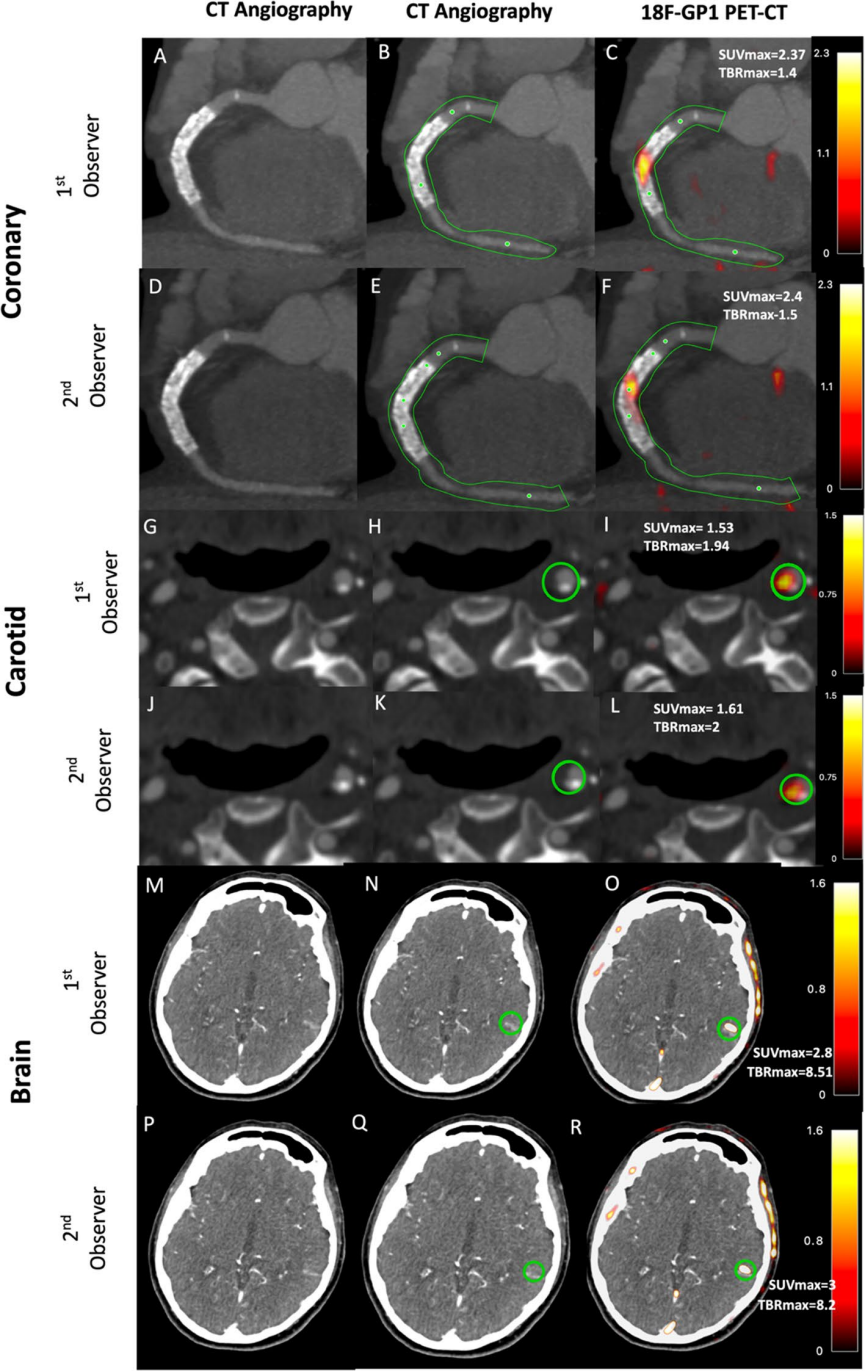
Quantitative ^18^F-GP1 positron emission tomography and computed tomography image analysis. Panels (A)–(F) show a patient presenting with inferior ST elevation myocardial infarct and receiving primary cutaneous intervention to the right coronary artery. CT angiography (A and D) with semiautomated centrelines placed to generate volumes of interest (B and E; green lines representing tubular region of interest). ^18^F-GP1 is seen within culprit right coronary artery and stented segment (C and F). Panels (G)–(L) show a patient presenting with left partial anterior circulatory stroke secondary to left carotid artery atherosclerotic disease. Carotid artery CT angiography (G and J) shows a mixed plaque disease in the internal carotid artery within the region of interest (H and K; green circle representing spherical region of interest). ^18^F-GP1 uptake seen associated with culprit plaque (I and L). Panels (M)–(R) show a patient with left partial anterior circulatory stroke with infarction seen in left temporal lobe on CT angiography (M and P) with the region on interest drawn (N and Q; green circle representing spherical region of interest). ^18^F-GP1 uptake is seen within area of infarction (O and R).
